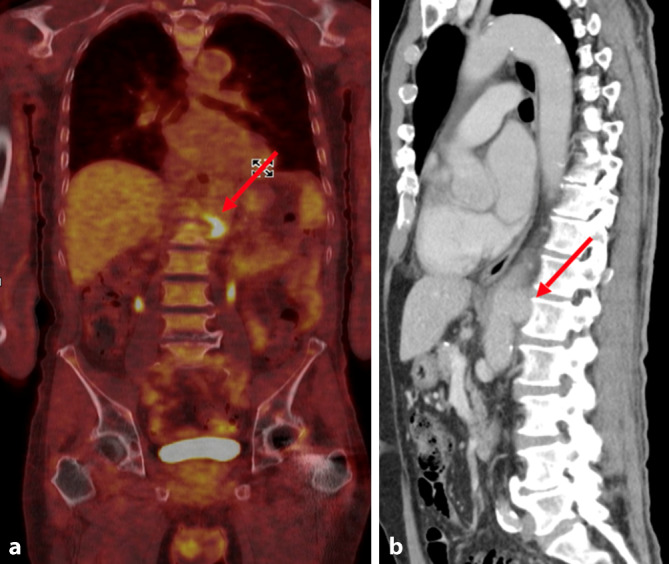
Ausgangsbefund des symptomatischen suprazöliakalen mykotischen penetrierenden Aortenulkus (PAU) – Fall 2. a „^18^F‑fluoro-D-deoxyglucose positron emission tomography“ (PET)/CT mit fokal erhöhtem Glukosestoffwechsel im Bereich eines Aneurysmas der Aorta abdominalis. b Computertomographische Aufnahme des PAUs (sagittale Rekonstruktion). Die *roten Pfeile* zeigen auf das Aortenaneurysma (Quelle: Klinik für Radiologie und Nuklearmedizin, Universitätsklinikum Magdeburg A. ö. R.)
